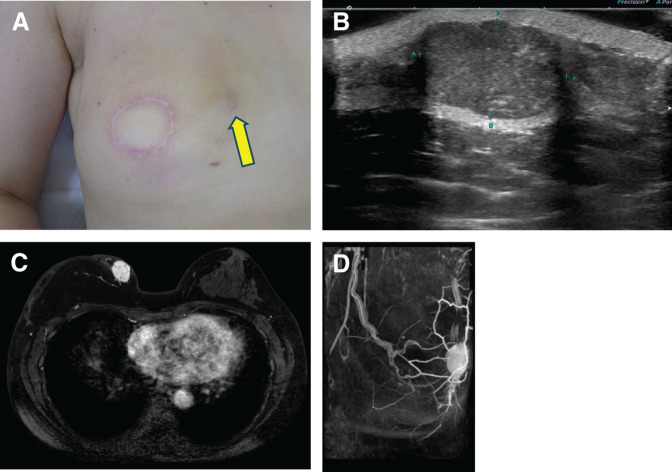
Physical examination revealed a 25-mm elastic hard mass in the upper inner region of the right breast, with thinning of the overlying skin. No palpable axillary lymphadenopathy was detected on the right side. The yellow arrow indicates the location of the tumor (A). US demonstrated a well-defined, irregularly shaped mass with internal hyperechogenicity, measuring up to 23-mm in maximum diameter, in the upper inner region of the right breast (B). Contrast-enhanced breast MRI demonstrated a 24-mm enhancing mass in the superficial aspect of the upper inner region of the reconstructed right breast (C, D).
